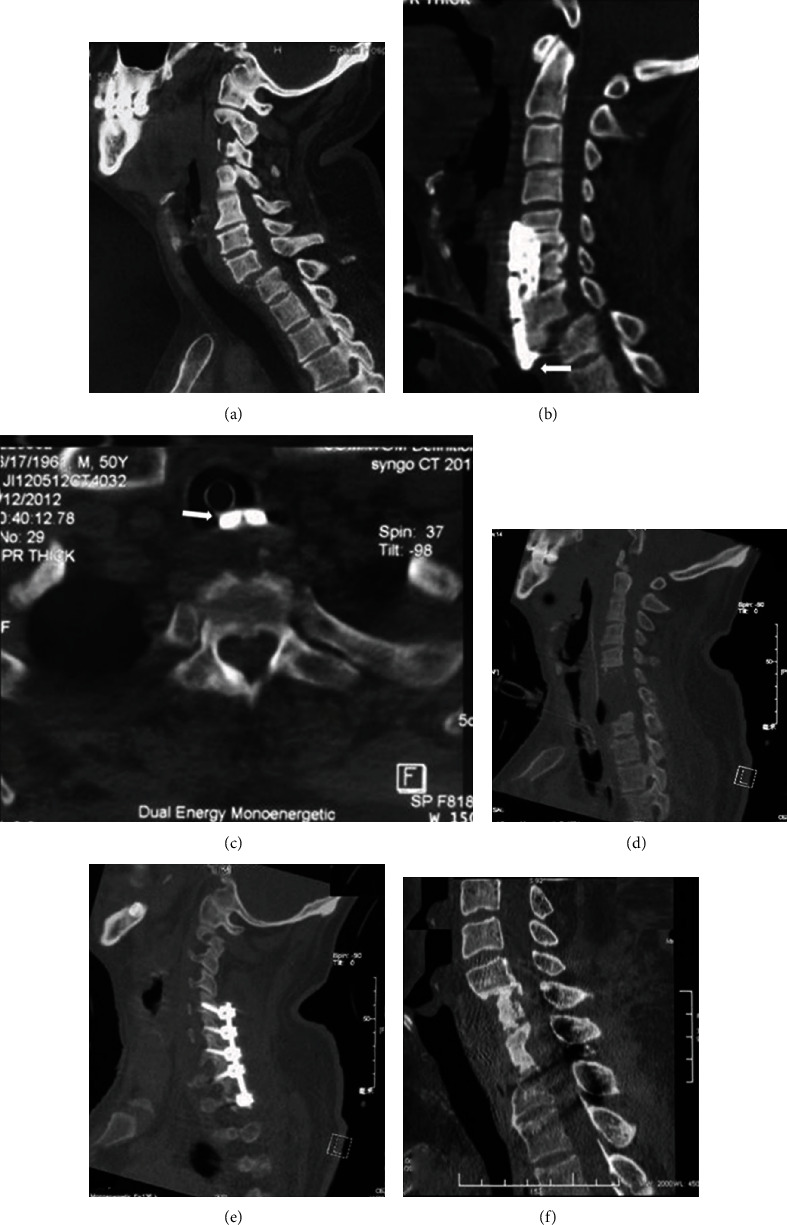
Images of case 2. (a) Preoperative sagittal CT reconstruction showing the anterior dislocation of C7; (b) postoperative sagittal CT reconstruction showing the failure of the instrumentation and the penetration of the distal end of the plate into the posterior tracheal wall (white arrow); (c) axial CT scan showing the penetration of the plate into the lumen of the trachea, with the plate in contact with the endotracheal intubation (white arrow); (d) sagittal CT reconstruction following the revision procedures demonstrating the restoration of the alignment of the cervicothoracic junction; (e) sagittal CT reconstruction following the revision procedures showing the posterior reduction and fixation from C5 to T2; and (f) sagittal CT reconstruction 24 months after the revision procedures showing the good maintenance of the alignment of the cervicothoracic junction.
